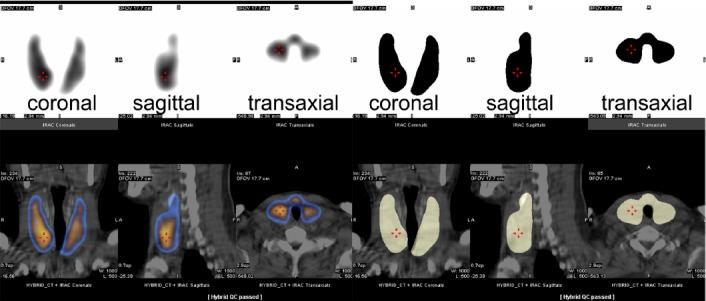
Simultaneously imaged slices of a diffuse enlarged thyroid in a patient with Graves’ disease (GD). Images on the top left show the pertechnetate image acquisition data including those of the coronal, sagittal, and transaxial slices. Images on the top right are the pertechnetate images processed using the SUV-shape scheme. Images at the bottom represent the fusion imaging of images in the top row and the corresponding low-dose CT images. The coronal slice shows the increased length and width of thyroid lobes, with both lobes extending from the superior border of thyroid cartilage to the superior border of clavicle. The sagittal slice shows the increased depth of the right lobe. The transaxial slice shows the enlarged isthmus of thyroid
